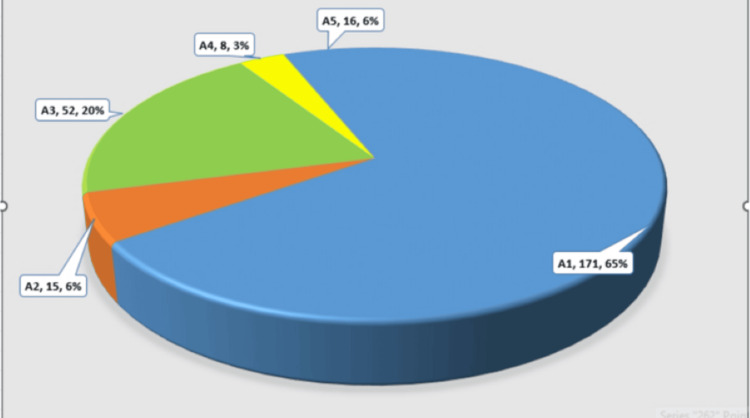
Total number of patients subjected to axillary ultrasound, n=262
